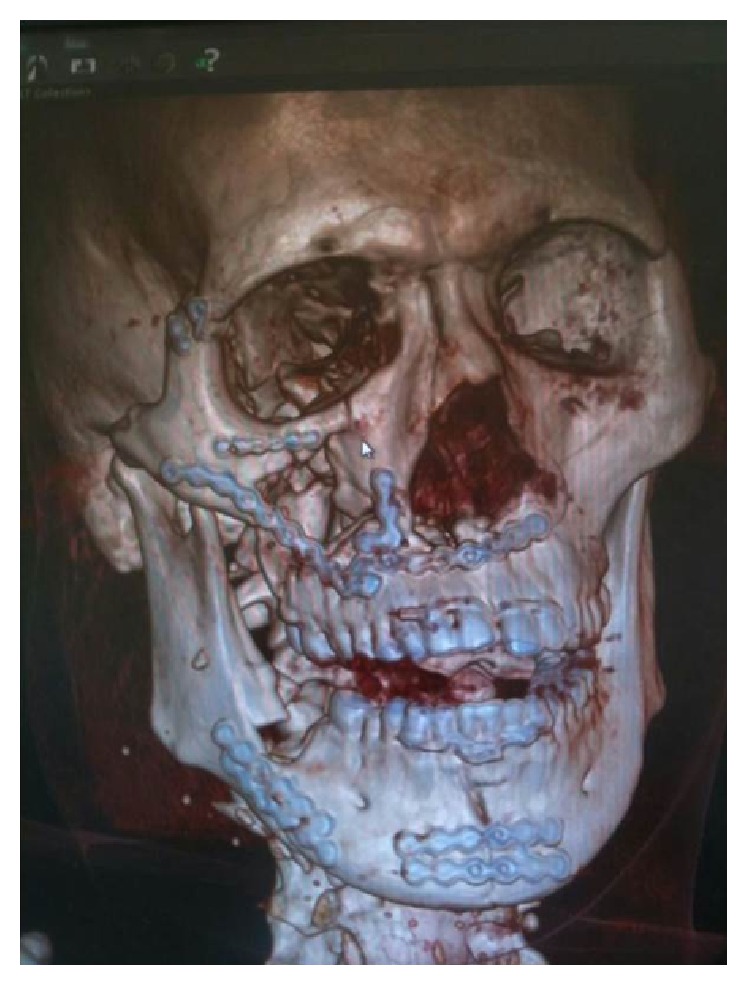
Postsurgical 3D CT scan.
